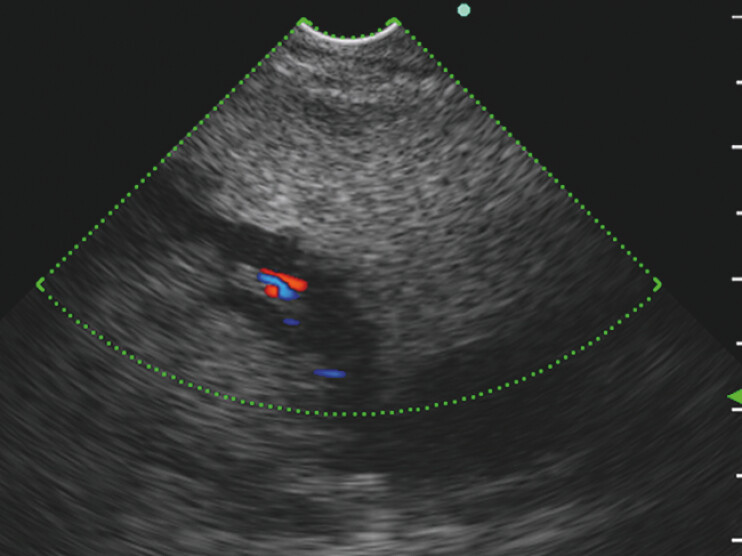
Left intrahepatic biliary branch depicted by forward-viewing curved linear echoendoscope (FV-CLS) from the residual stomach. Endoscopic ultrasonography-guided biliary drainage (EUS-BD) via the residual stomach was likely achieved; however, we hesitated to perform a puncture because the left intrahepatic biliary branch was narrow (3.1 mm in diameter).
